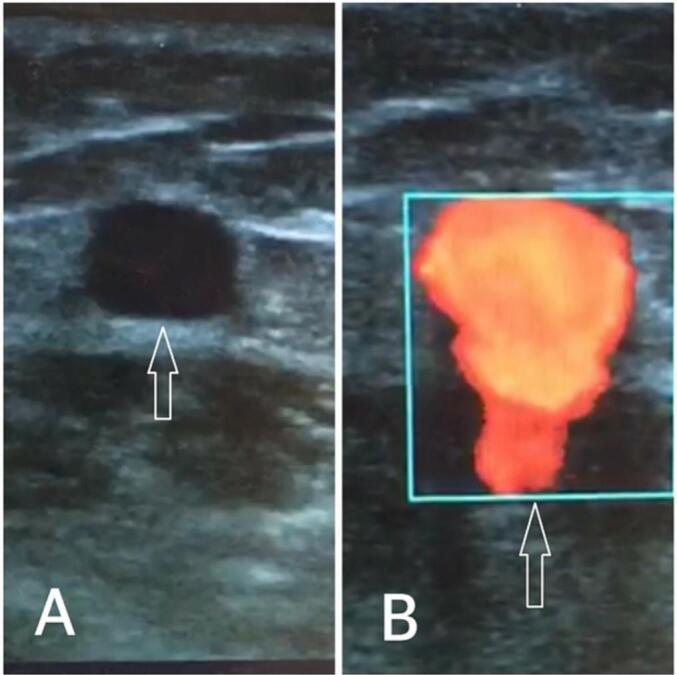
A: Great saphenous vein in color Doppler sonography; B: Arterial flow in great saphenous vein.
